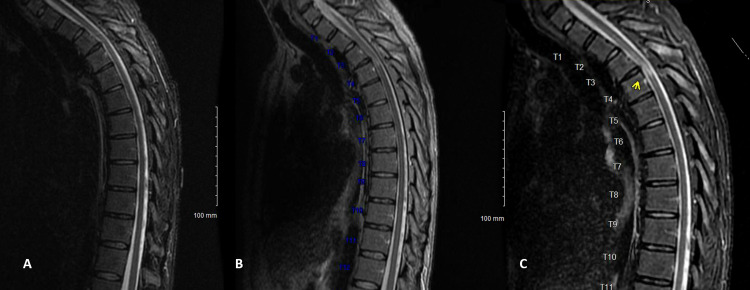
Serial Throracic MRI Sequences Short T1 inversion recovery sequencing sagittal MRI views of the patient’s thoracic spine at A) one week, B) one month, and C) two months after the thoracic radiofrequency ablation procedure. Cord signal changes were not present at one week but were seen in the one-month study and had slightly increased at two months (arrow indicating location of cord signal changes).
